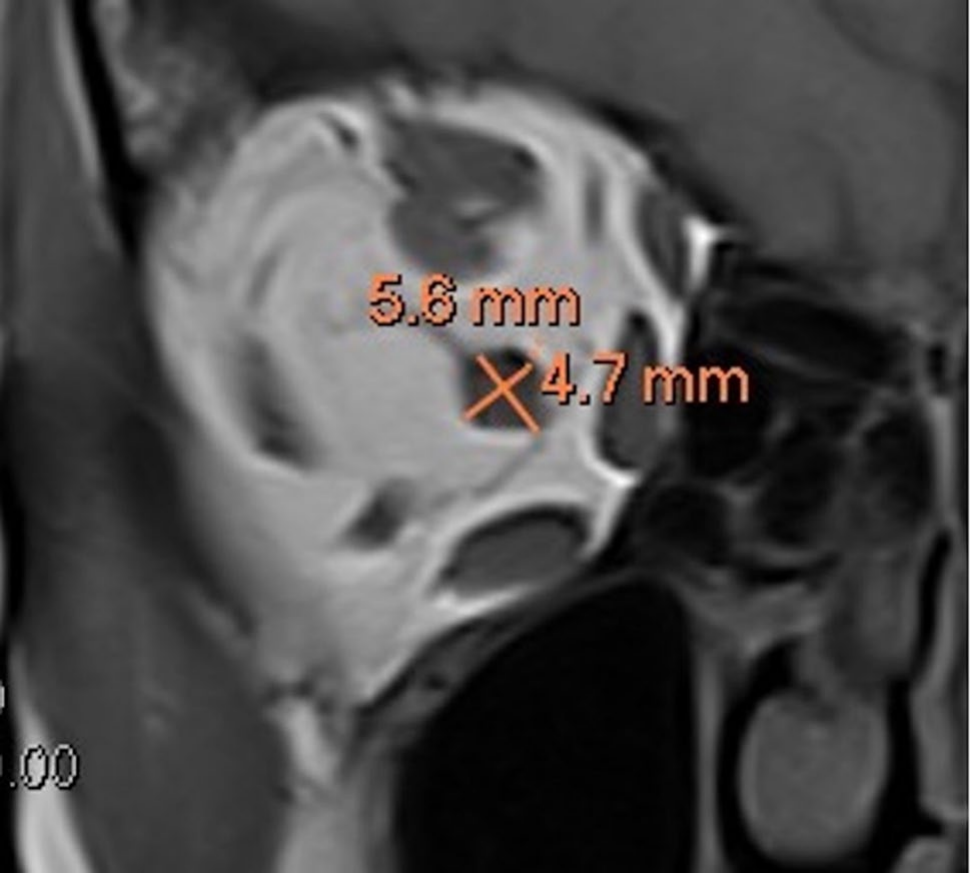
Coronal T1-weighted magnetic resonance imaging showing the short-axis dimension, measured perpendicular to the long-axis, of the optic nerve sheath
